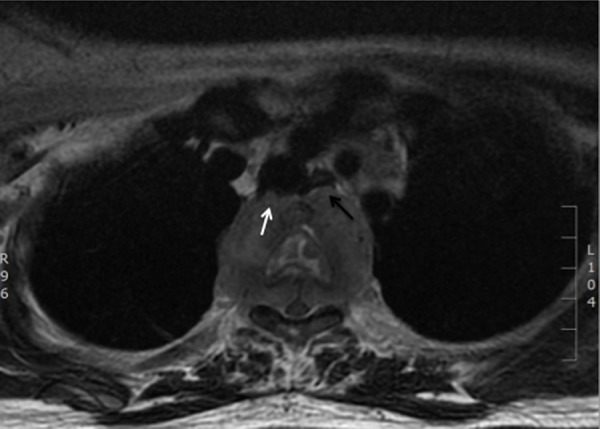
Axial T2 fat suppressed MR image at the level of T3 showing a paravertebral mass extending into the spinal canal causing spinal cord displacement and compression. The mass has also indented the posterior wall of the trachea (white arrow) and displacing the esophagus anteriorly (black arrow).
